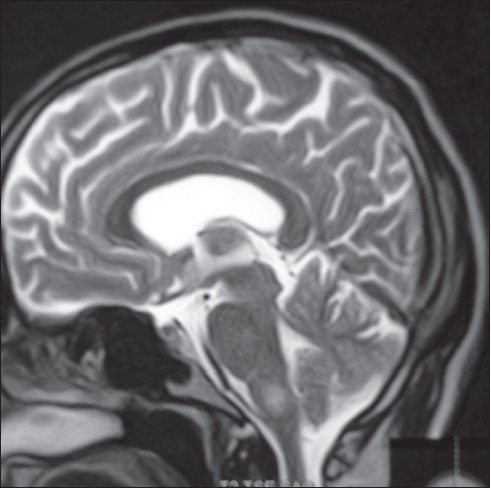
One week after a febrile illness, a 55 year old man developed neurogenic dysphagia accompanied by ataxia and drowsiness. Clinical examination revealed ophthalmoplegia, cerebellar signs and sluggish deep tendon reflexes. MRI of the brain showed a single hyperintense lesion in the pons, On T2 W images which moderately enhanced with contrast. He rapidly recovered following intravenous steroids
